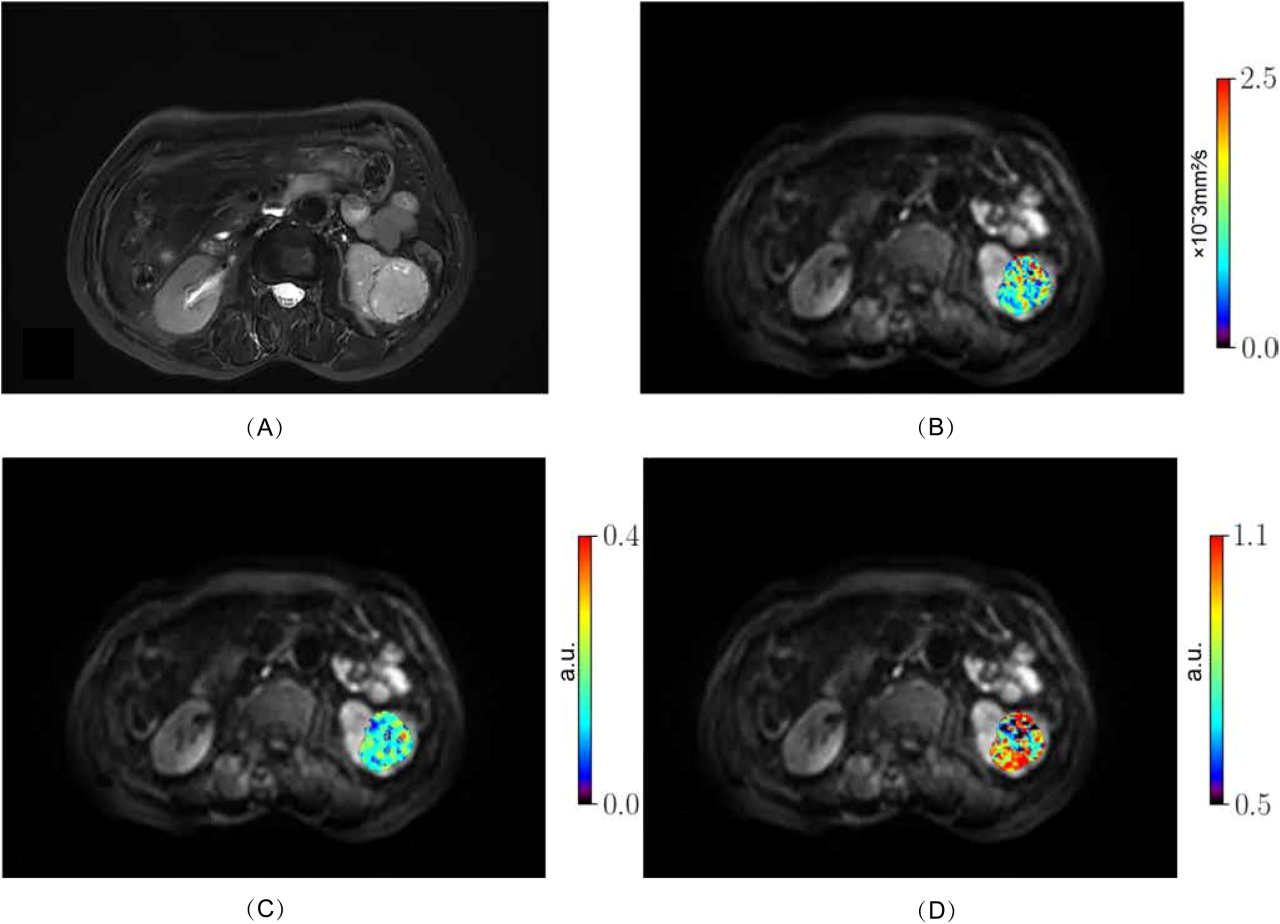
Low-grade ccRCC (WHO-ISUP grade 2) in the left kidney of a 76-year-old woman. (A), axial fat-suppressed T2-weighted image; (B–D) MR images with quantitative MAD maps: (B), D_h_ values; (C), f_r_ values and (D), α_h_ values. ccRCC, clear cell renal cell carcinoma; MAD, multimodal apparent diffusion model.
